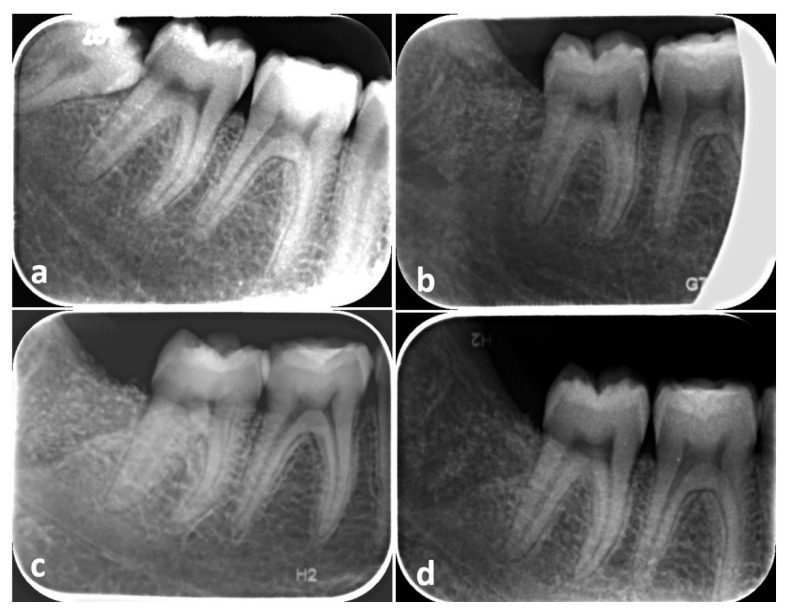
Radiographic images showing the treatment sequence at an experimental site: (a) Presurgical periapical radiograph; (b) 15 days, (c) 90 days and (d) 180 days after socket grafting.
